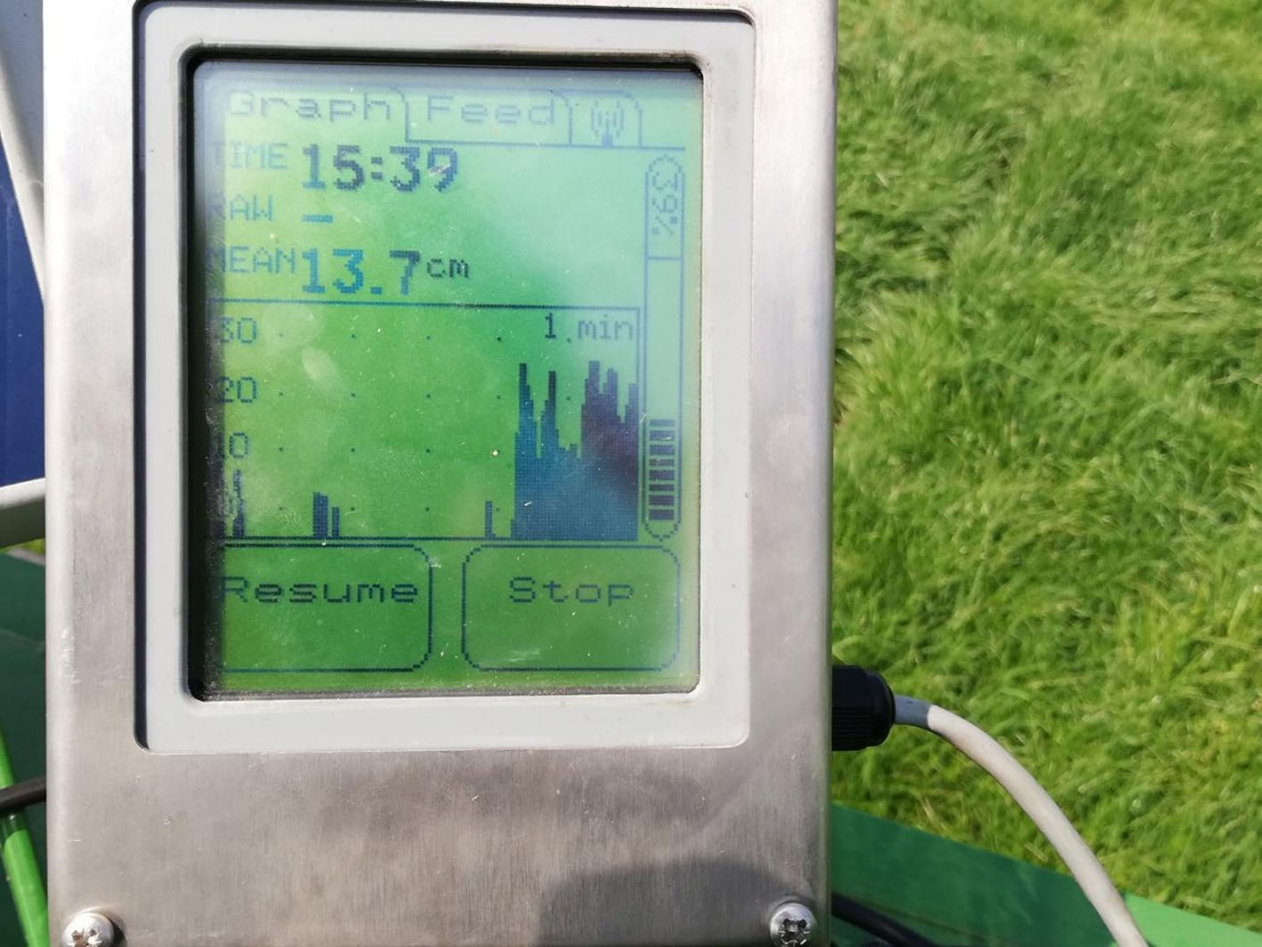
Ultrasonic sensor, Pasture Reader, for plant height measurement. http://pasturereader.com.au/
